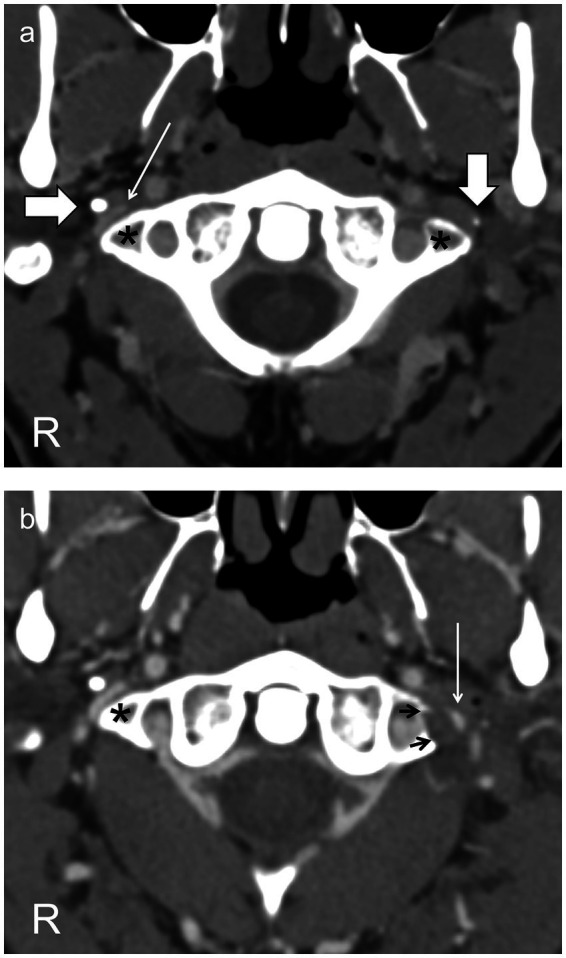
Axial CT scans through the C1 vertebra following intravenous contrast (A) before surgery show the jugular vein on the right side (thin arrow) markedly narrowed between the styloid process (horizontal thick arrow) and the C1 transverse process (asterisk) and on the left side compressed into invisibility between the styloid process (vertical thick arrow) and the C1 transverse process (asterisk). (B) After resection of the left styloid and C1 transverse process (resection margin, black arrows), the left jugular vein (thin arrow), though still narrowed, is now visible. The right jugular vein is unchanged, as expected.
